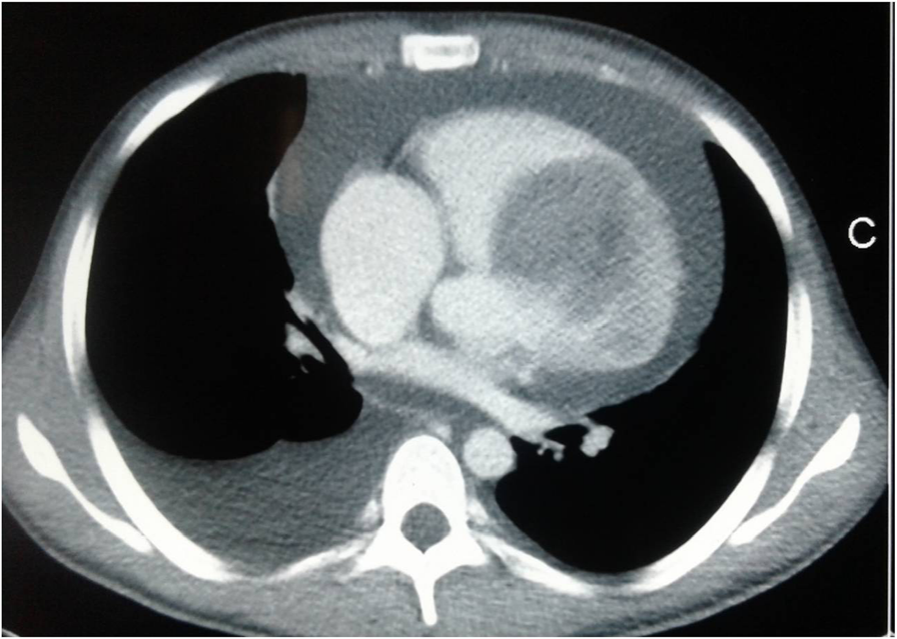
Chest computed tomography scan showing right ventricular mass, pericardial effusion, and pleural effusion
